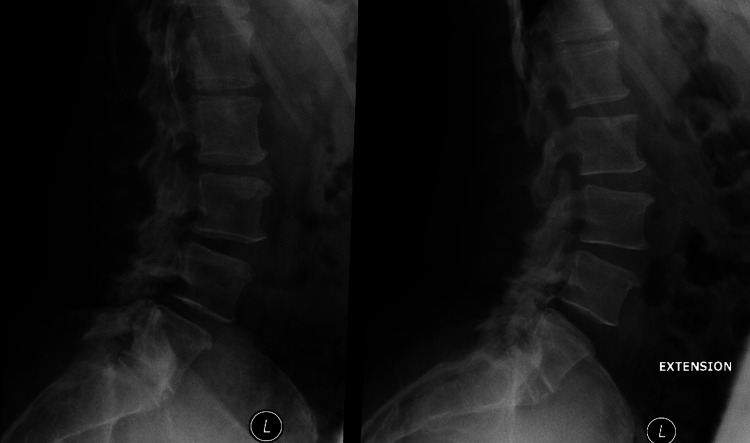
Preoperative flexion and extension of lumbar spine (Left image) Lateral flexion x-ray of the lumbar spine with grade 1 spondylolisthesis that reduces with extension (right image).
